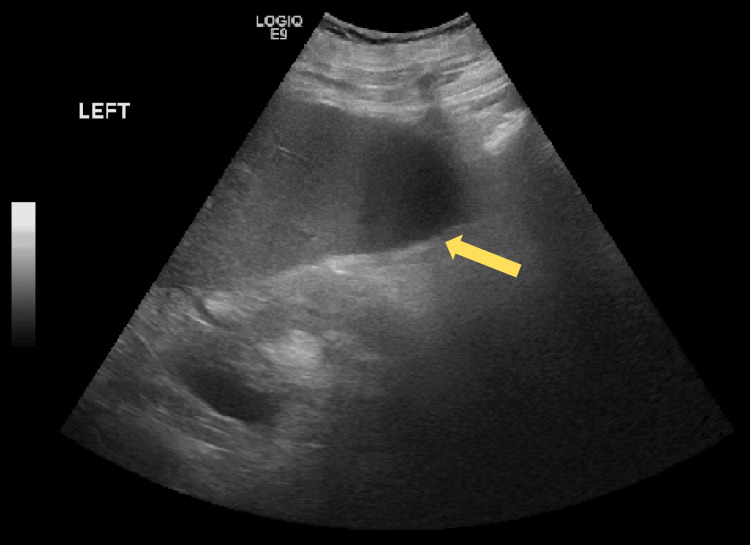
Renal ultrasound revealing ascites (yellow arrow).
